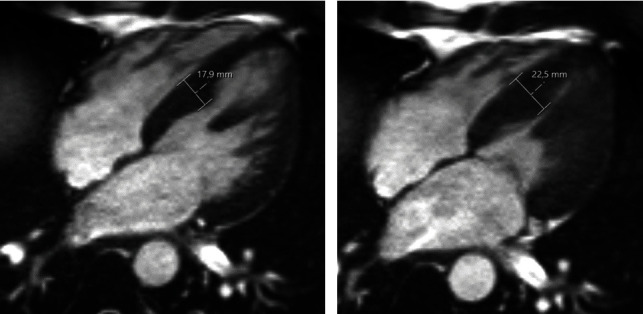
Cardiac MRI of the patient's brother (1.5 Tesla, cine sequence). (a) End-diastolic septal myocardial hypertrophy with maximum measured septum thickness of 17.9 mm. (b) End systolic septal myocardial hypertrophy with maximum measured septum thickness of 22.5 mm.
